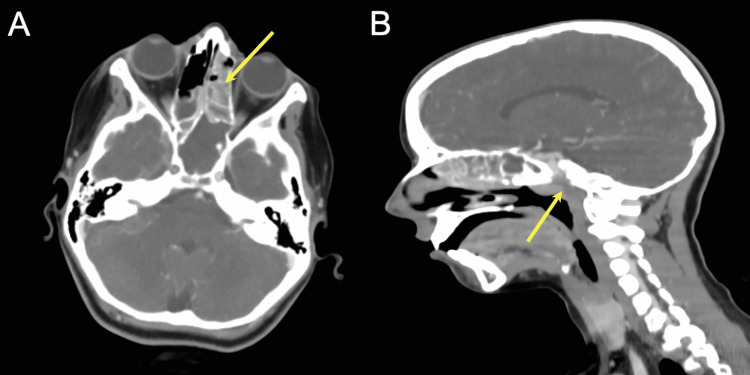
Initial CT scans showed polysinusitis in the axial image (A) and parapharyngeal abscess in the sagittal image (B).
